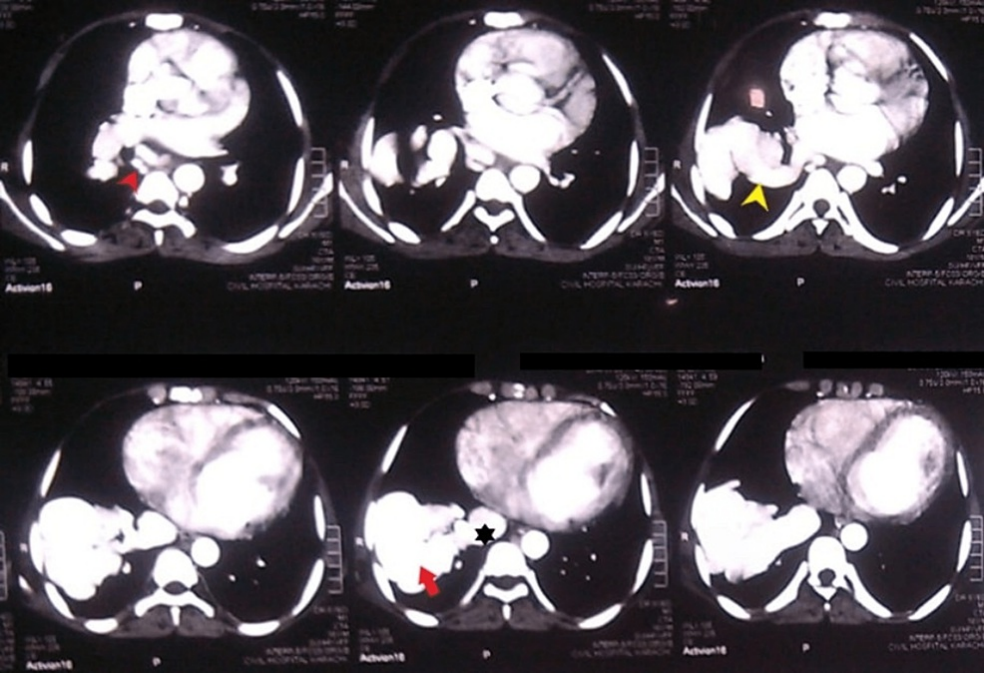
Contrast-enhanced computed tomography (CT) angiogram of the chest It demonstrated a serpiginous hypervascular mass in the lower lobe of the right lung measuring 7.0 x 8.1 x 5.3 cm.
